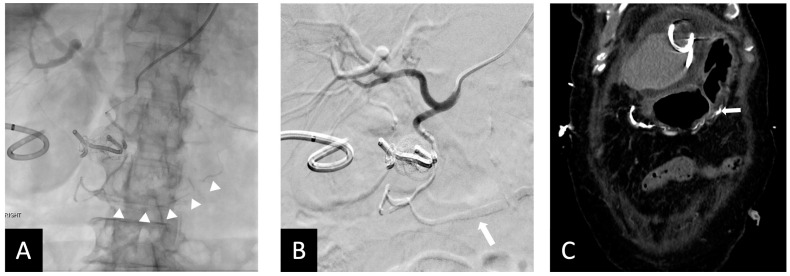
Fifty-five y.o male with bleeding duodenal ulcer. (A) Ta-OCE embolization of the GDA without using a backstop coil in distal GDA/proximal RGEA. (Arrow shows extension of Ta-OCE in the mid- to distal RGEA). (B) Post-GDA embolization DSA, demonstrating adequate GDA embolization and better visualization of Ta-OCE cast in the mid- and distal RGAE (arrow). (C) CT scan showing Ta-OCE extension into the distal RGEA post-embolization (arrow). Bleeding was successfully treated in this patient with no sign of bowel ischemia or injury. Image obtained from patient’s chart. Source: NK.
